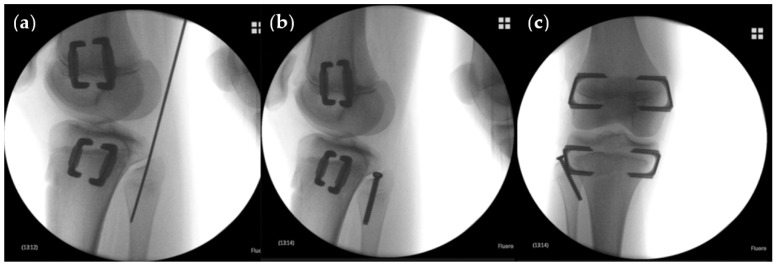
Operative technique of proximal fibular epiphysiodesis. (a) Intraoperative lateral radiograph of proximal fibular epiphysiodesis with a cannulated fully threaded screw implanted in a K-wire guided technique concomitantly with distal femoral and proximal tibial temporary epiphysiodesis in a 12-year-old boy. (b) Intraoperative lateral radiograph of same patient after implantation. (c) Intraoperative anteroposterior radiograph of same patient after implantation.
